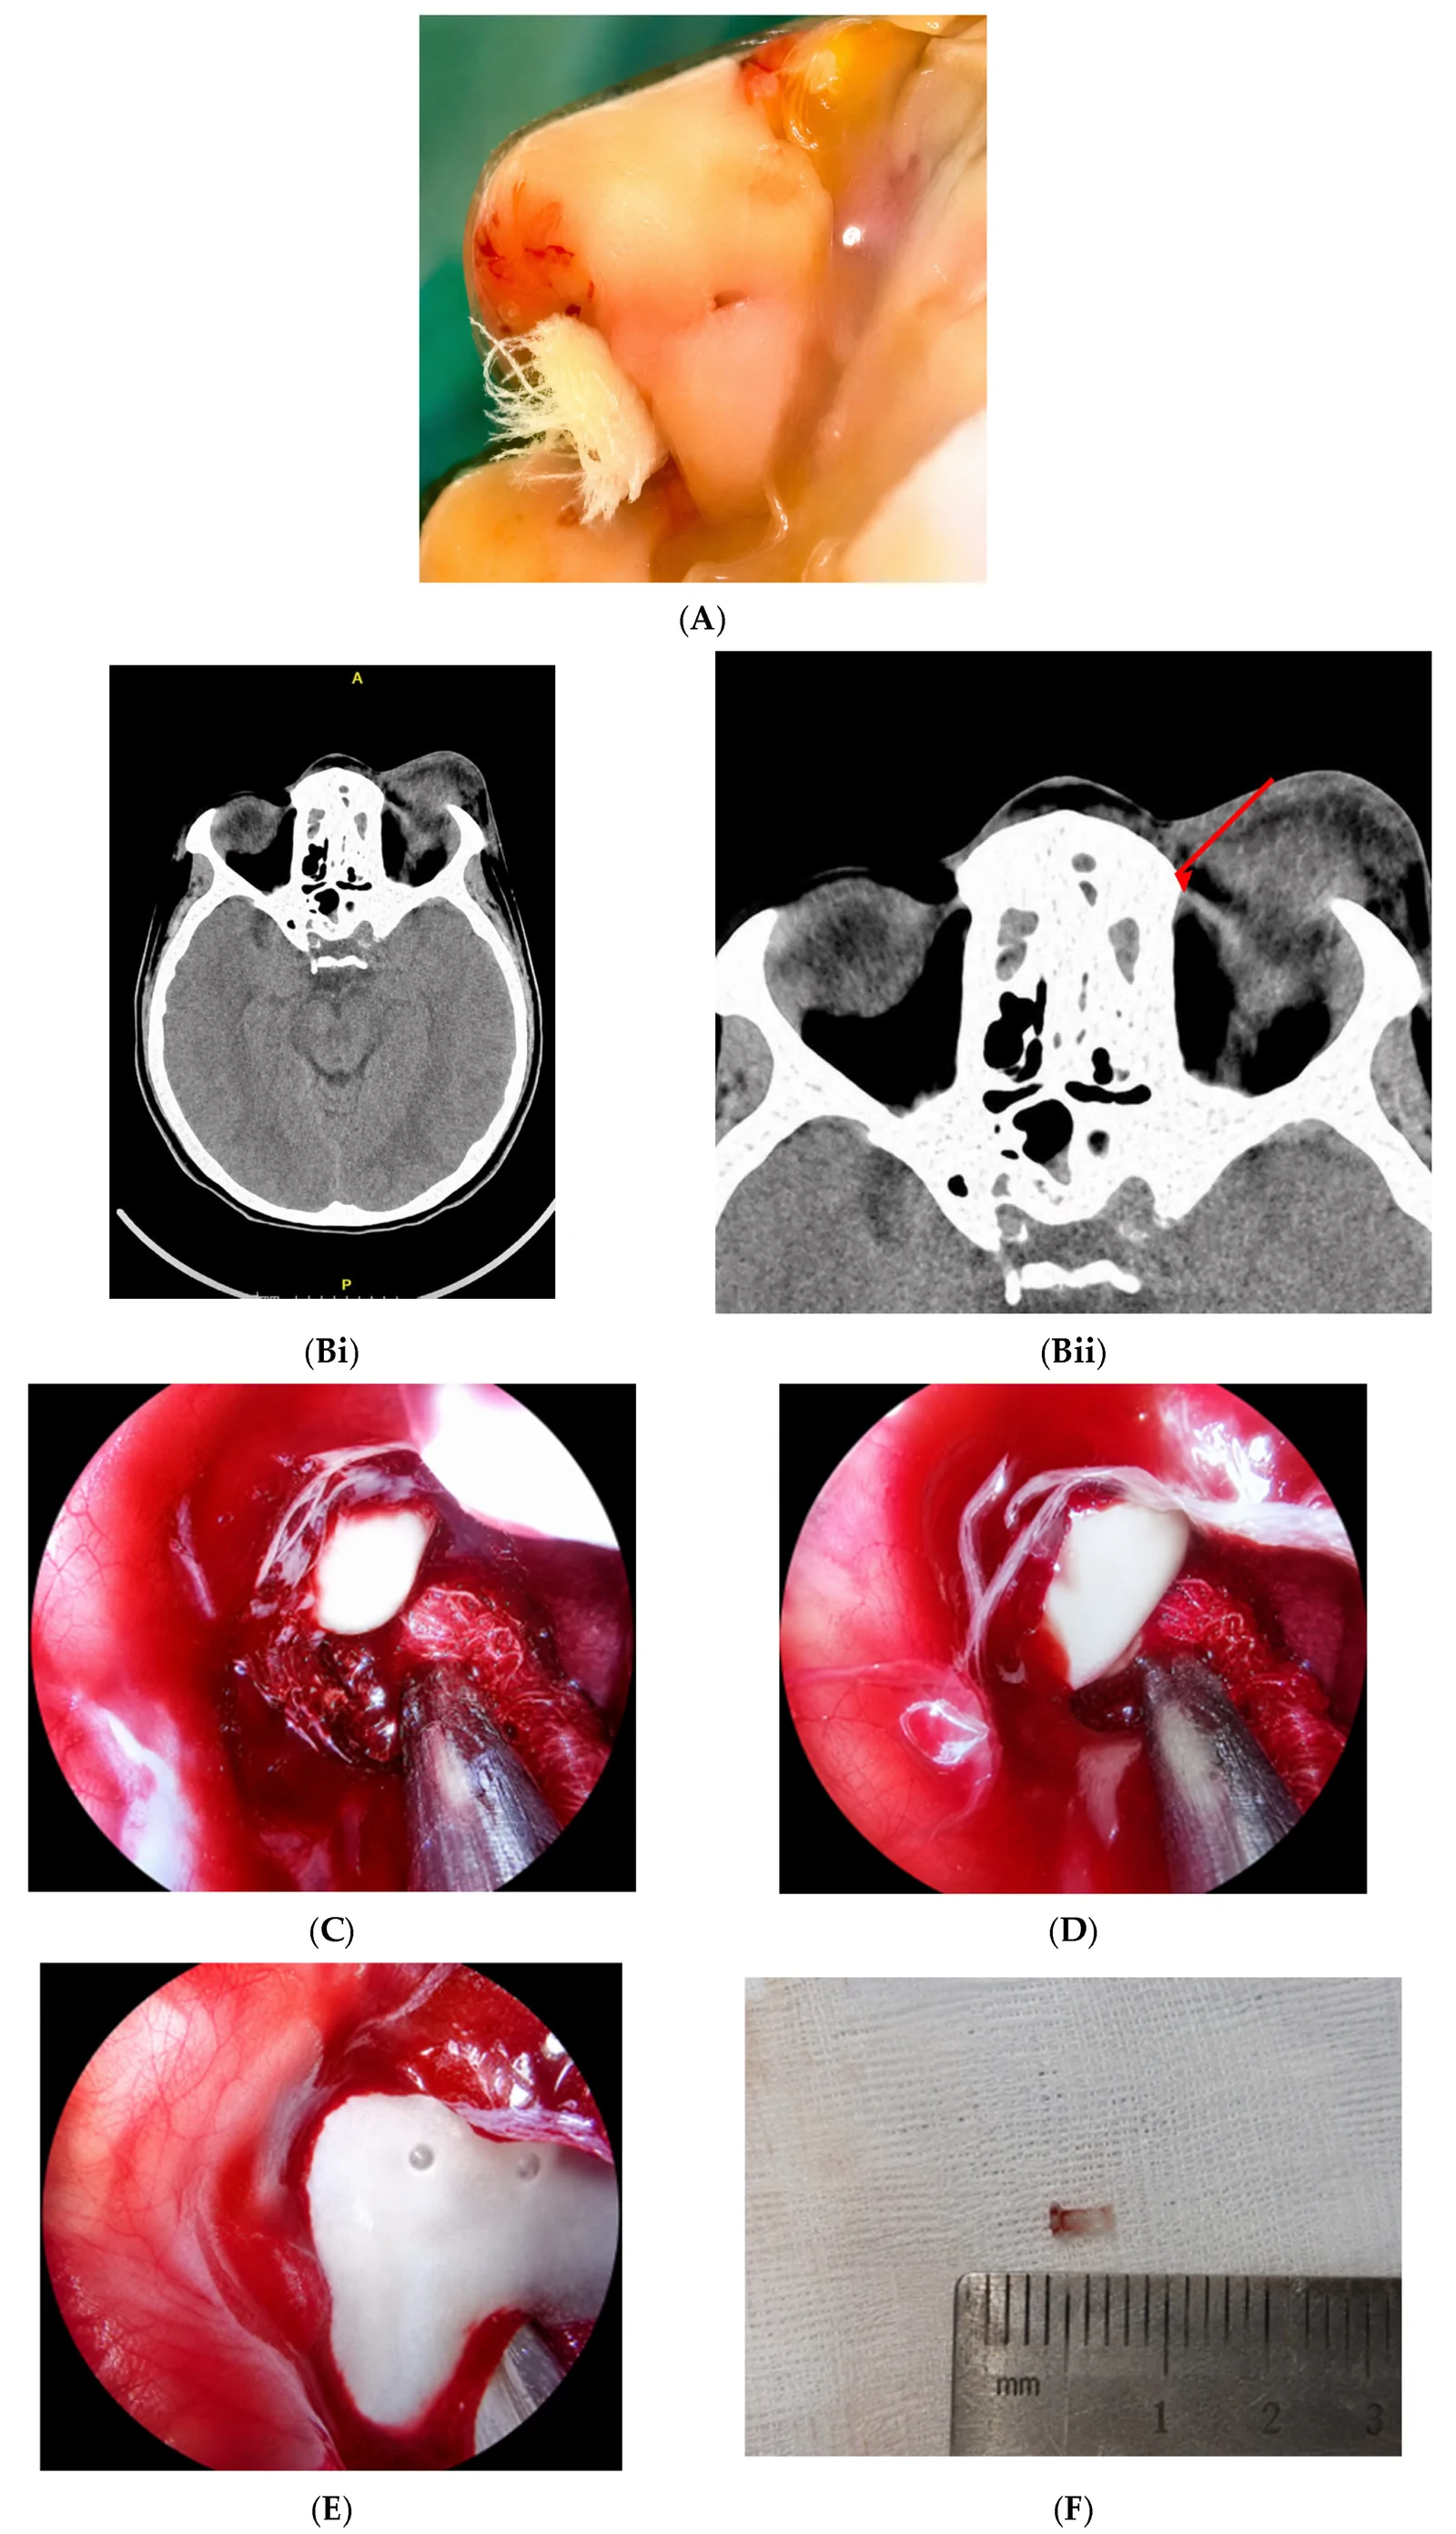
Orbital complication secondary to retained intranasal foreign body. (A) External nasal piercing site. (Bi) Axial CT showing left ethmoiditis and medial orbital subperiosteal abscess, (Bii) Close-up of the (Bi) Axial CT showing a more focused view of the subperiosteal abscess (red arrow). (C–E) Sequential endoscopic views during drainage and debridement. (F) Removed plastic locking component.
